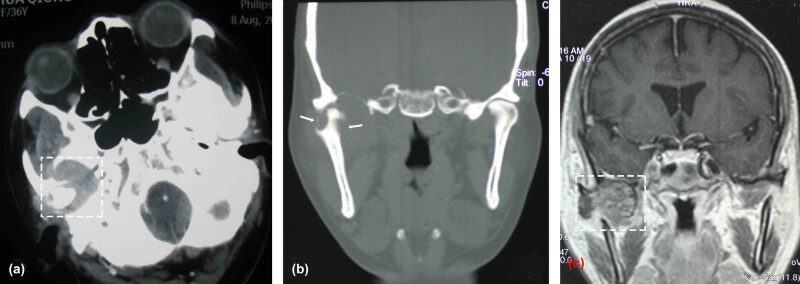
The CT scan shows a 3.5 cm^2^ × 4.0 cm^2^ expansive neoplasm located in the right TMJ region (a, horizontal plane, white rectangular region), with an expansile osteolytic process that was eroding the mandibular condyle (b, coronal plan, white arrows). MRI results confirmed an irregular neoplasm surrounding the mandibular condyle with an unclear joint space (c, coronal plan, white rectangular region).
